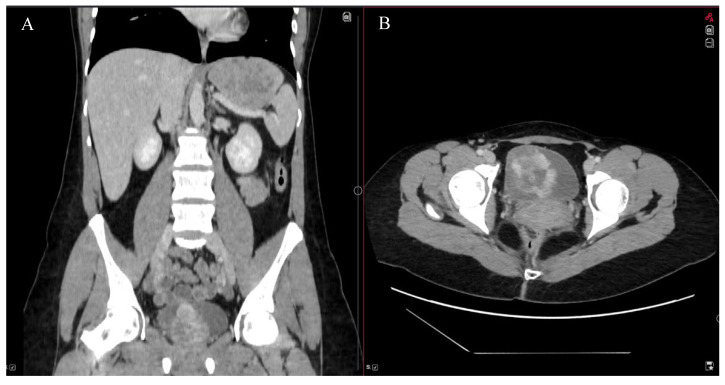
Frontal (A) and axial (B) CT scan of abdomen and pelvis showed a 5.3 × 5.1 × 5.8 cm mass in the urinary bladder, which accumulated contrast material.
